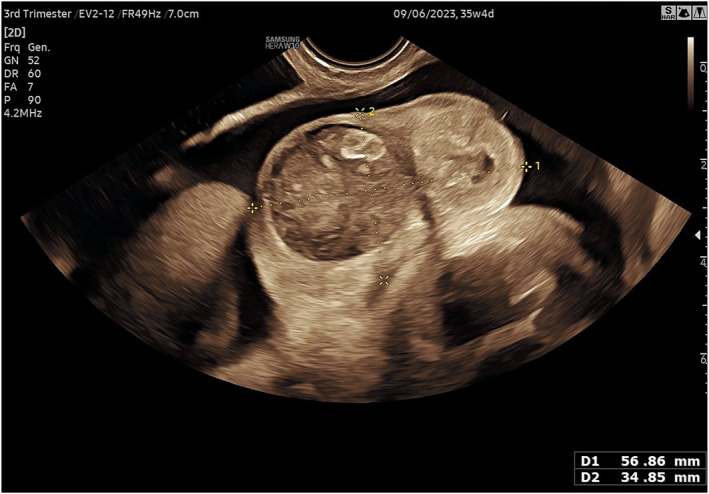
Ultrasound image (Transabdominal scan) of the scrotum in a fetus with MPO.
